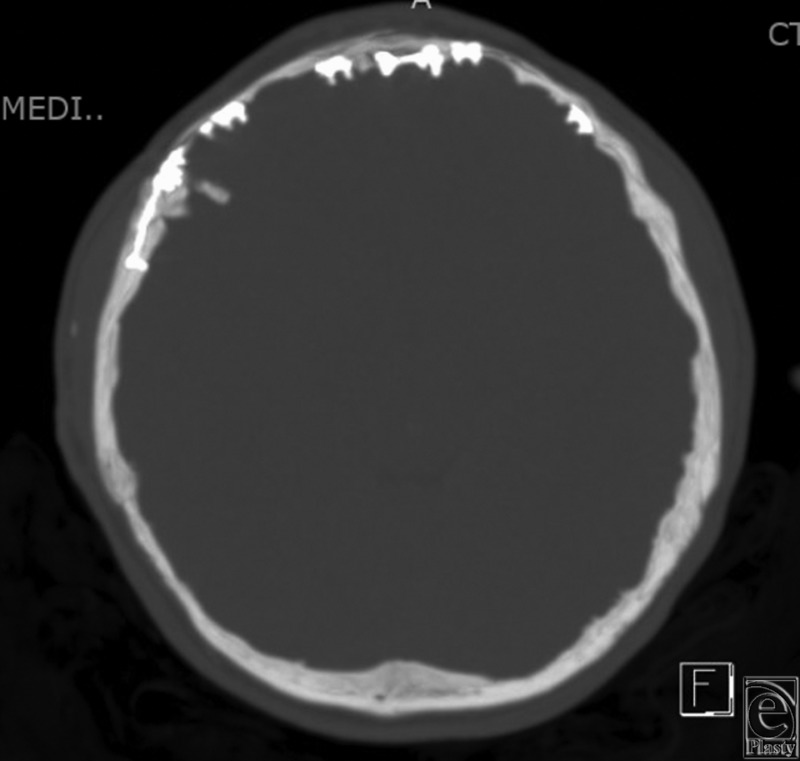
Axial computed tomographic scan of intracranial extension of hardware.
